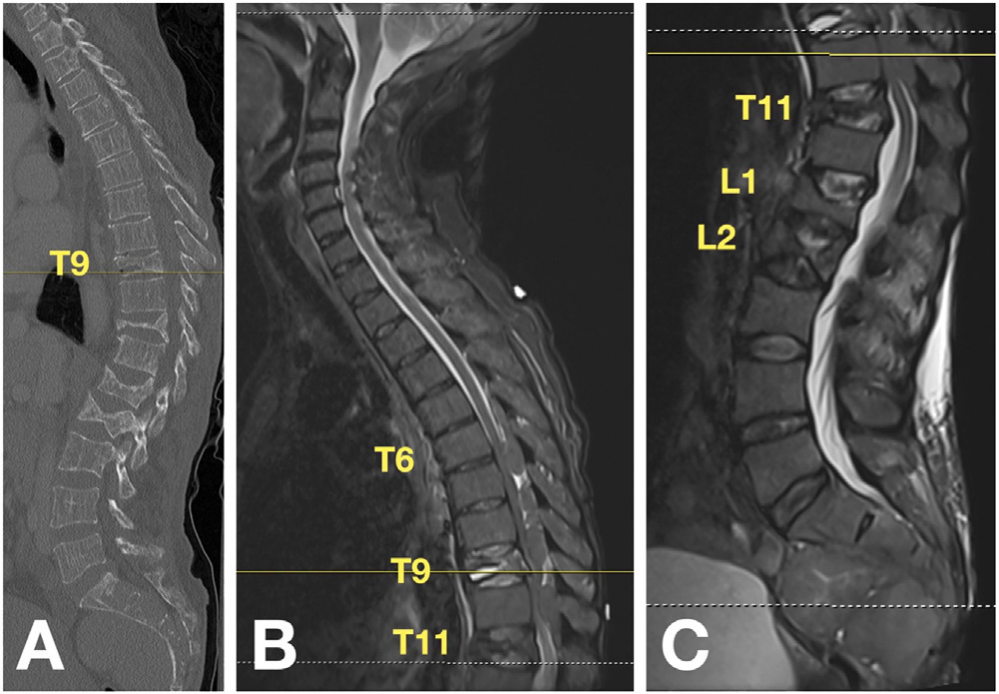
Seven months after initial presentation, CT (A) demonstrating acute T9 compression fracture with vacuum phenomenon. Thoracic (A) and lumbar (B) MRI re-demonstrating compression fractures at T11, L1, and L2 and the dorsal extradural masses from T6 to T10.
